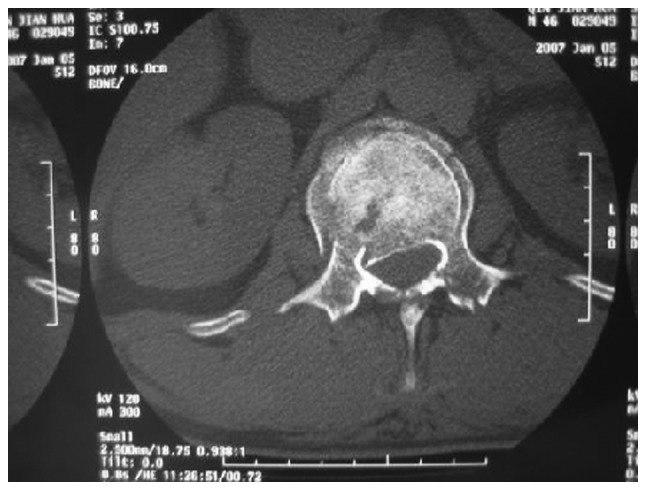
Preoperative CT scanning image of a 45-year-old male.
